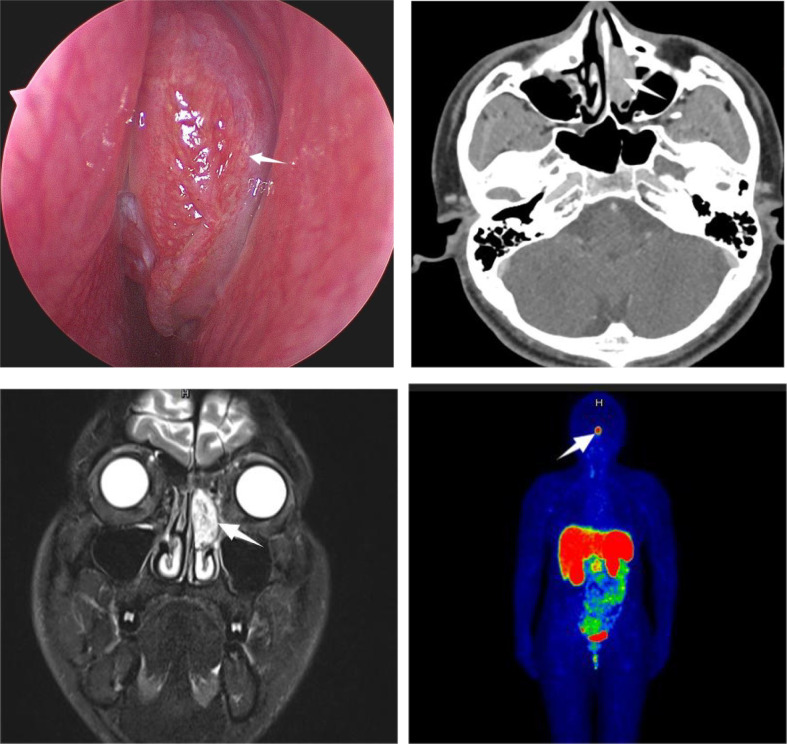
Typical imaging findings (case 4) (Endoscopy/CT/MRI/PET, white arrow: tumour).
